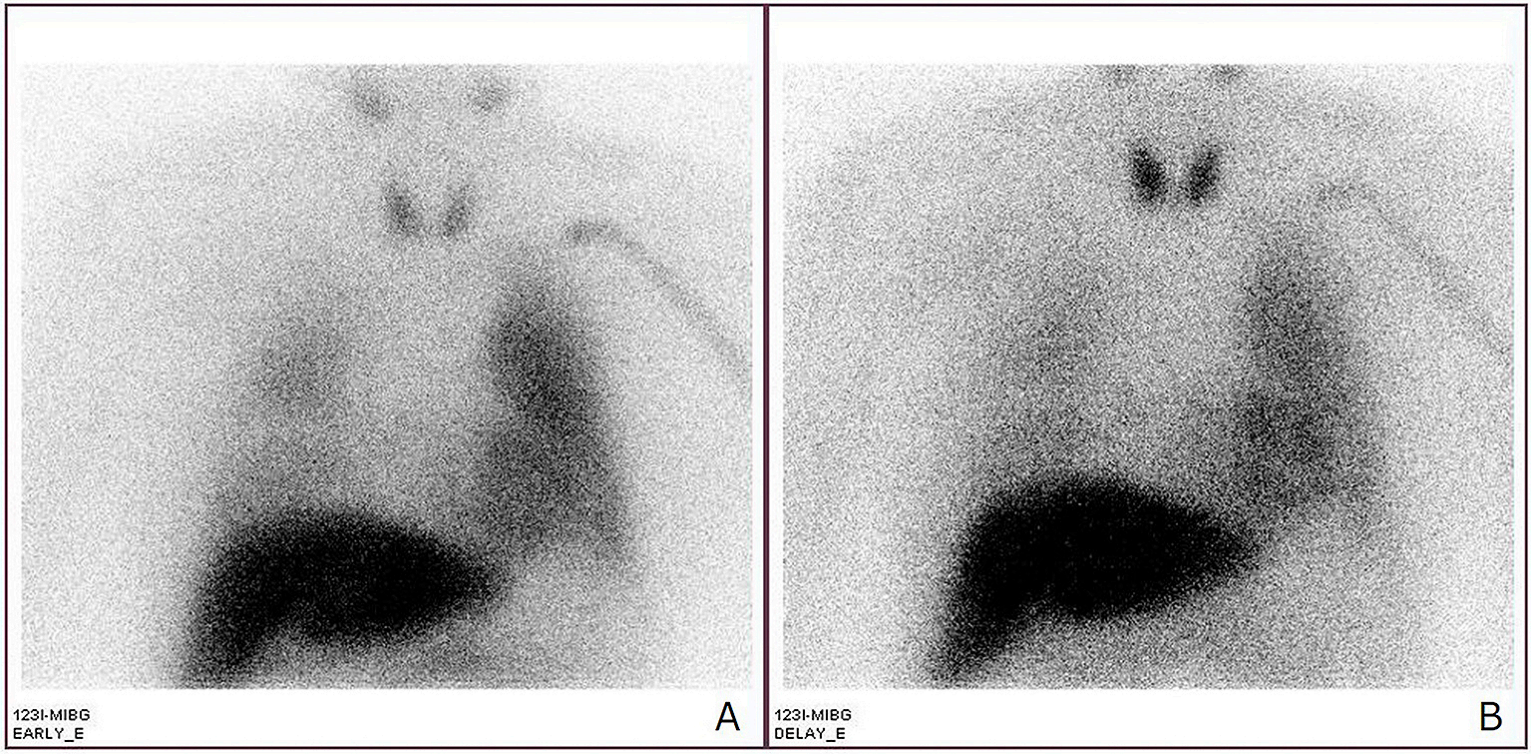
Cardiac [^123^I]-metaiodobenzylguanidine scintigraphy showed a normal early-phase heart-to-mediastinum ratio (2.50; reference range, 1.9-3.0) and an elevated washout rate (37.25%; reference range, 0%-34%) [(A) Early phase, (B) Delayed phase].
